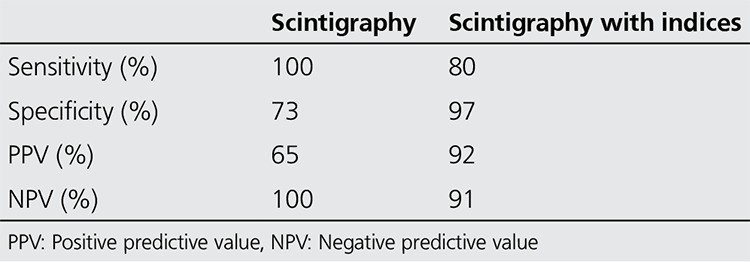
Results of scintigraphy and index values in diagnosis of sacroiliitis
